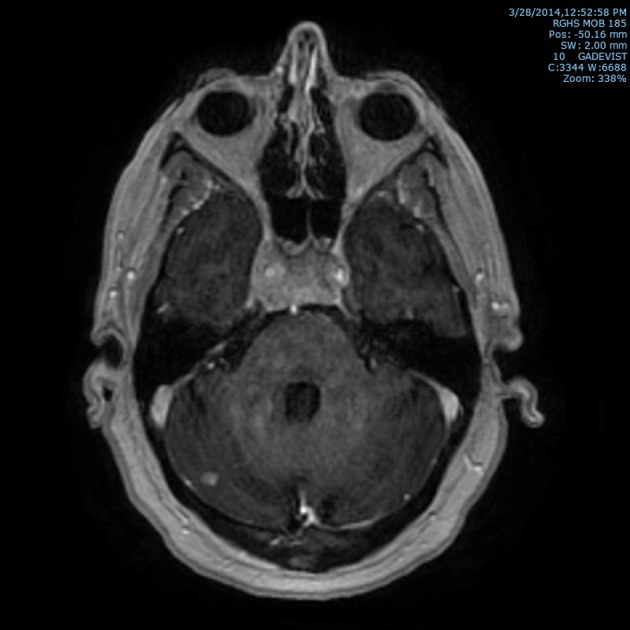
T1 post-contrast axial MRI image of brain showing marrow signal abnormality within the clivus with an expansile mass with soft tissue component in the cavernous sinus and right cerebellar metastasis.
